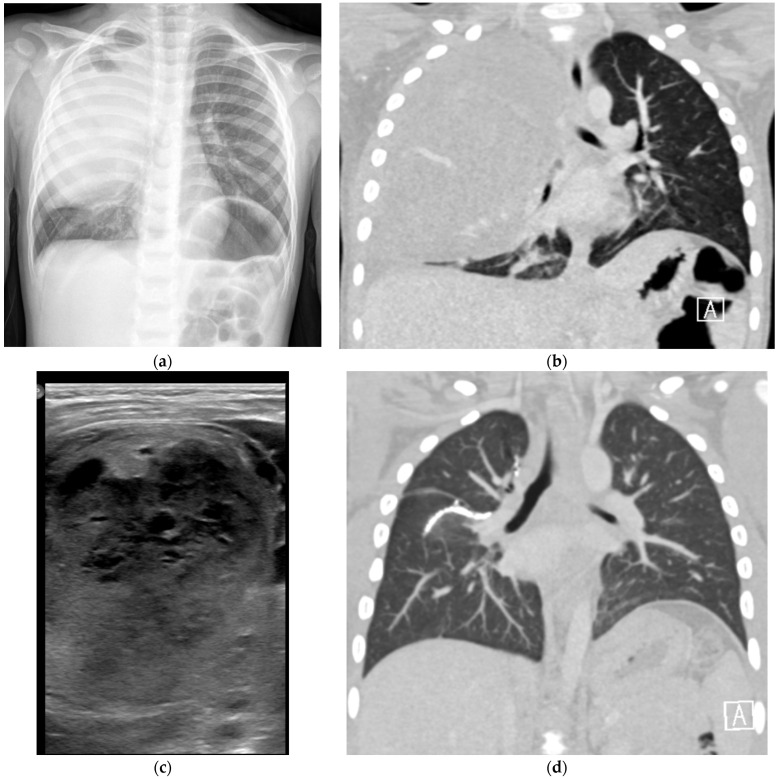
Six-year-old boy with progressive shortness of breath, fatigue and weight loss. (a) Chest X-ray: Opacification of the upper two thirds of the right upper hemithorax by a pleural-based lesion. There is a slight shift of the trachea to the left, and a caudal shift of the right diaphragm. (b) Coronal image of CTA showing large aberrant vessels within the lesion. (c) Sonographic gray-scale high-resolution image of the lesion (linear array transducer) shows the cystic/solid nature of the lesion. (d) Coronal image of CTA at the end of treatment, showing no evidence of recurrent disease.
